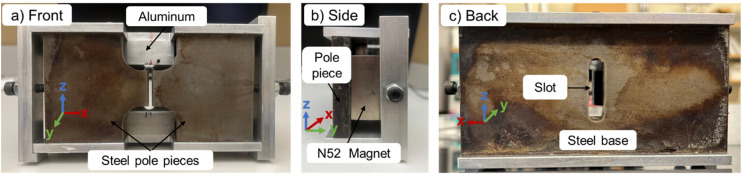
The magnetic separation system. (a) Front view shows the steel pole pieces that conduct the magnetic flux across the air gap and the aluminum frame used to assemble the pieces. (b) Side view shows how the magnets are located between the steel pieces. (c) Back view shows the slot that provides X-ray access to the sample.
